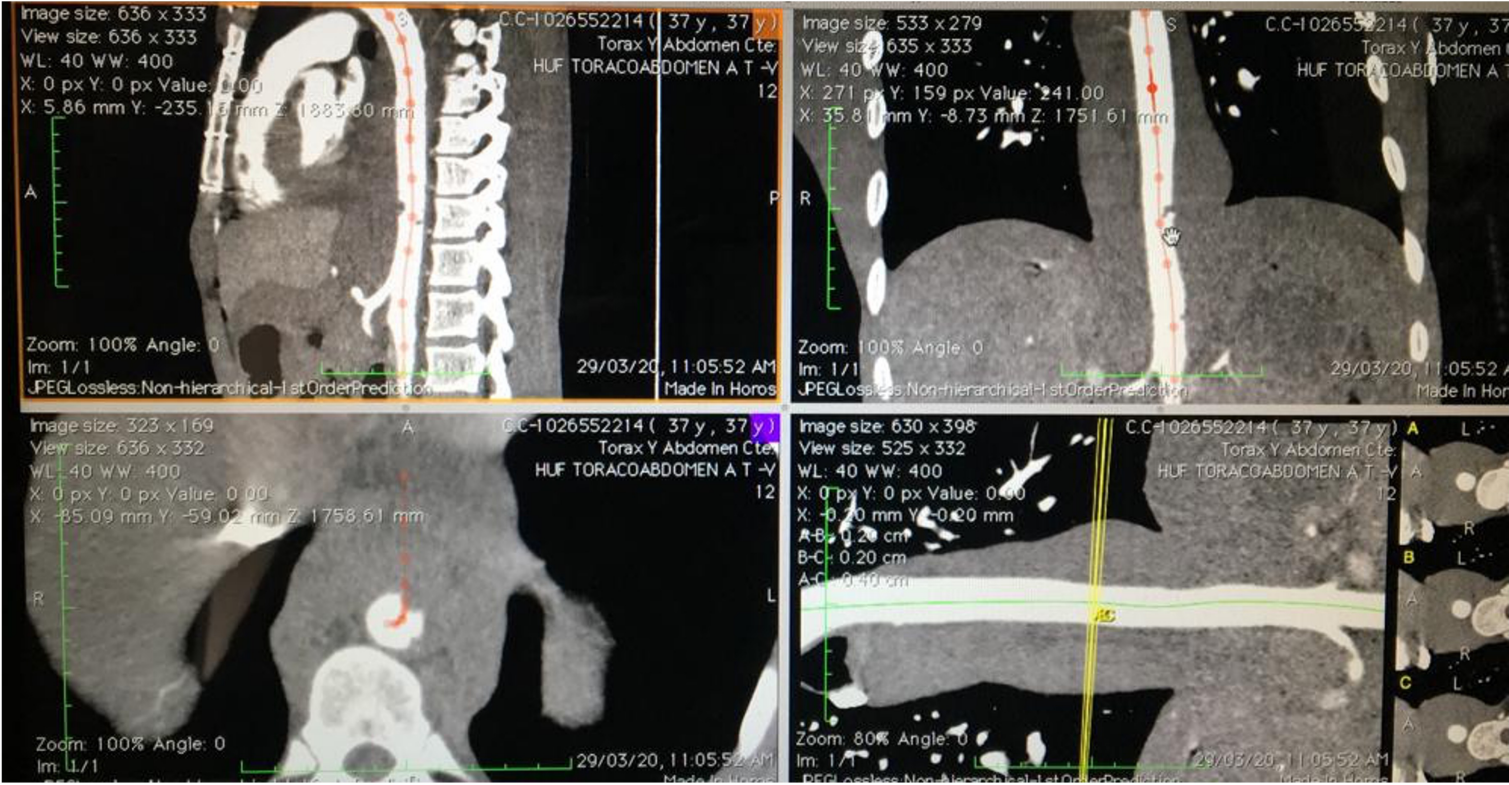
CT preoperative planification, measurement of the aorta.
